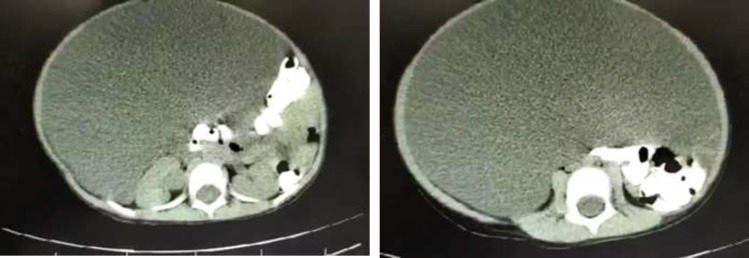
A: CT scan transversal view of the mass (mesenteric cystic lymphangioma). A) The appearance of the lesion
